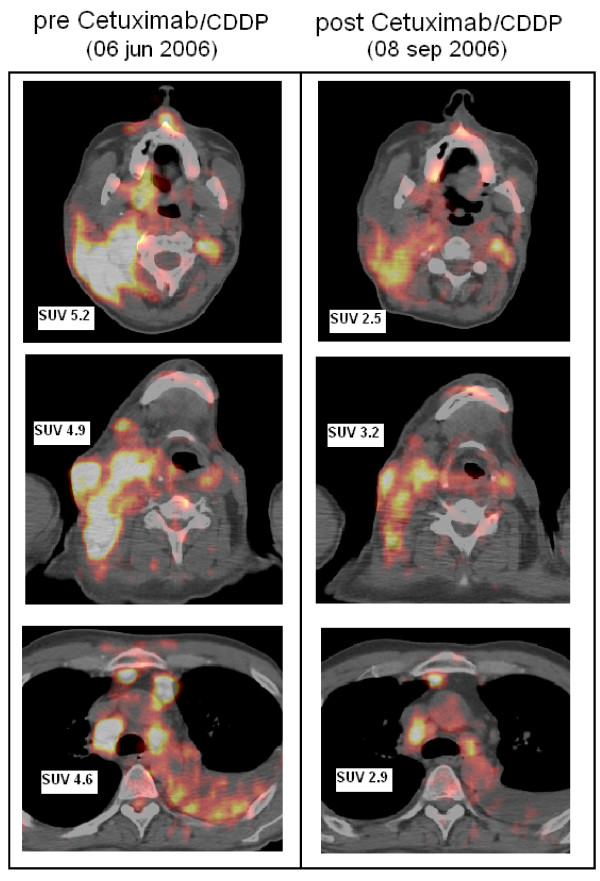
Total body computed tomography-positron emission tomography with [^18^F]fluorodeoxyglucose before (left panel) and after (right panel) treatment. Metabolically active neoplastic disease is located by high standardized uptake value of tracer: maximum standardized uptake value values are reported for each lesion before and after treatment.
